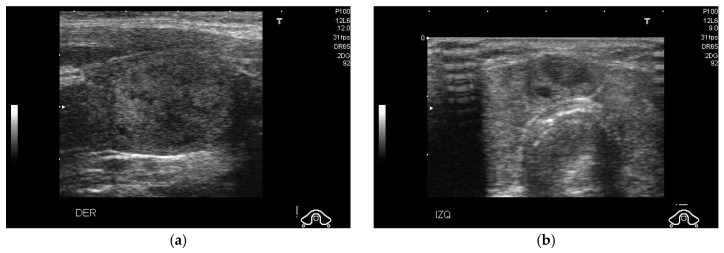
Example of captured ultrasound thyroid images: (a) a benign case, and (b) a malign case.
